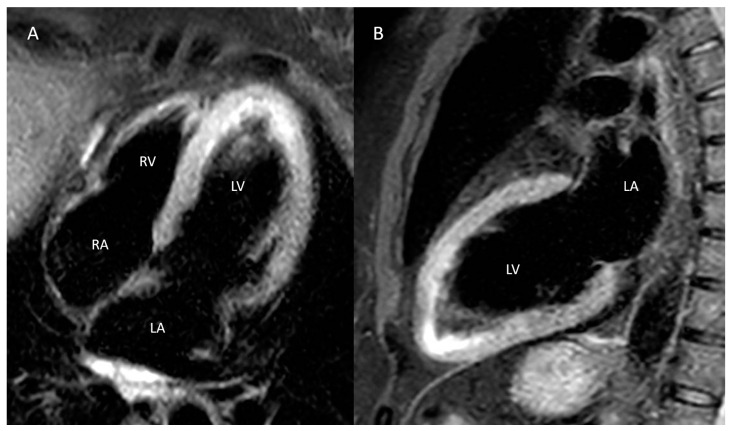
Cardiac magnetic resonance imaging: T2-weighted sequence showing the presence of diffuse edema of the apical segments in both the 4-chamber (A) and 2-chamber (B) long-axis views. LA: left atrium; LV: left ventricle; RA: right atrium; RV; right ventricle.
